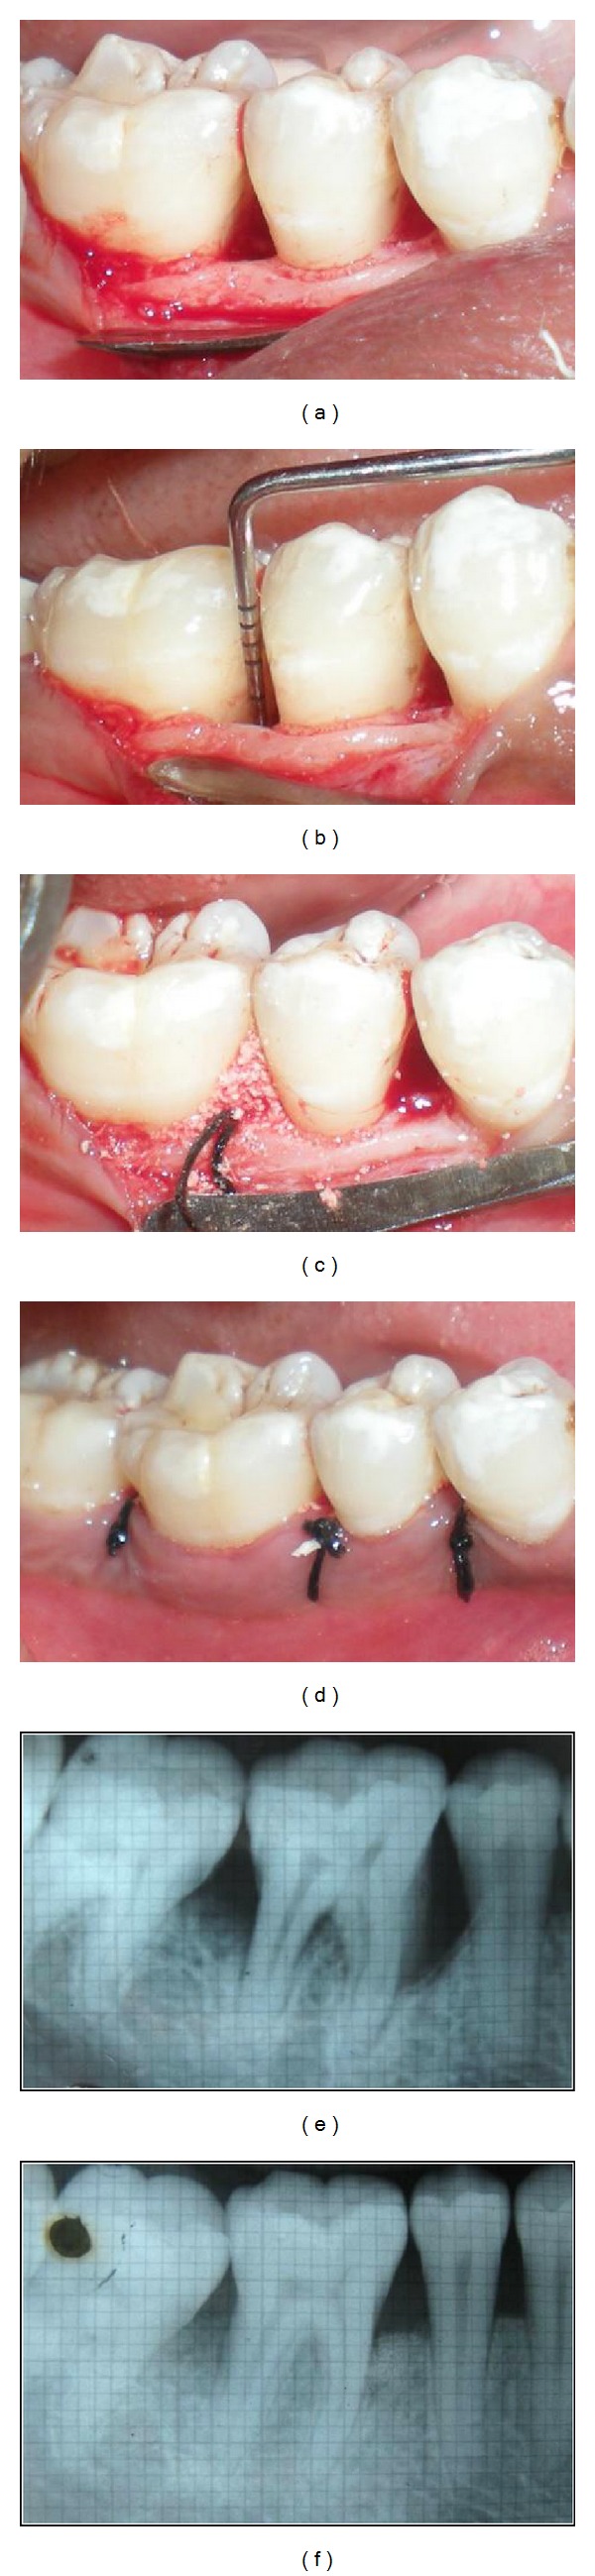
(a) Preoperative view. (b) Configuration and depth of defect. (c) Placement of rh-PDGF-BB in combination with *β*-TCP. (d) Suturing. (e) Preoperative radiograph. (f) Postoperative radiograph.
